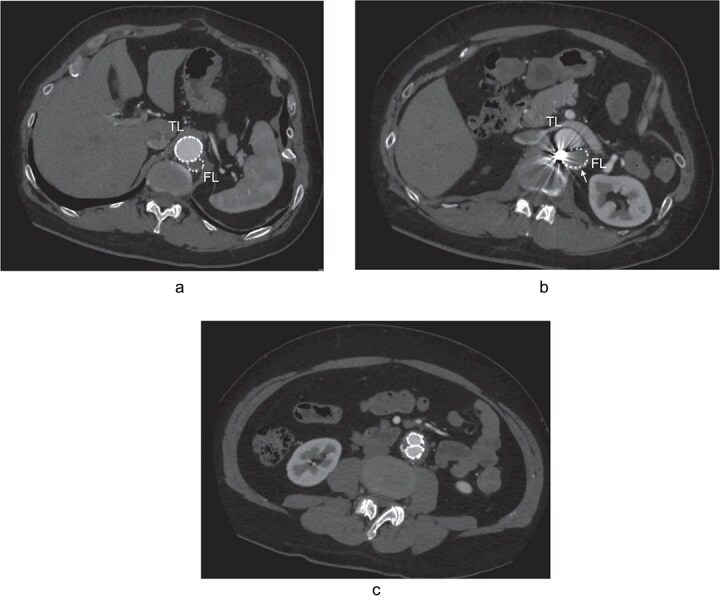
The postoperative contrast-enhanced CT axial images 3 years after the procedure showed no contrast inflow into the FL, and the reduced diameter of post-dissection thoracoabdominal aortic aneurysm at thoracic level (a), renal artery level with FL stent graft (b; white arrow), and abdominal level (c). TL: true lumen; FL: false lumen.
